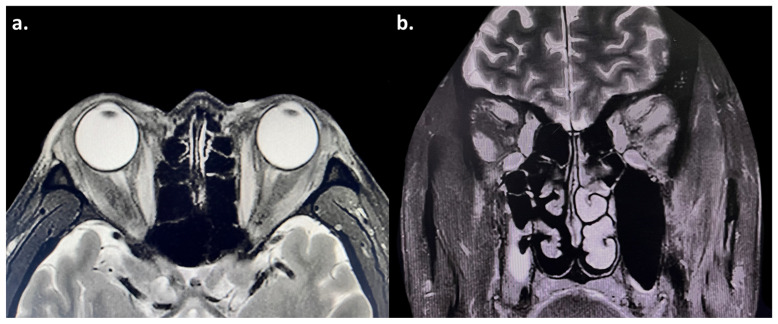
(a,b) Magnetic resonance imaging (MRI) showed enlargement of the bilateral medial rectus, superior rectus, superior oblique, inferior rectus, and lateral rectus muscles, with hyperintensity on T2-weighted images. The bilateral optic nerves were normal.
